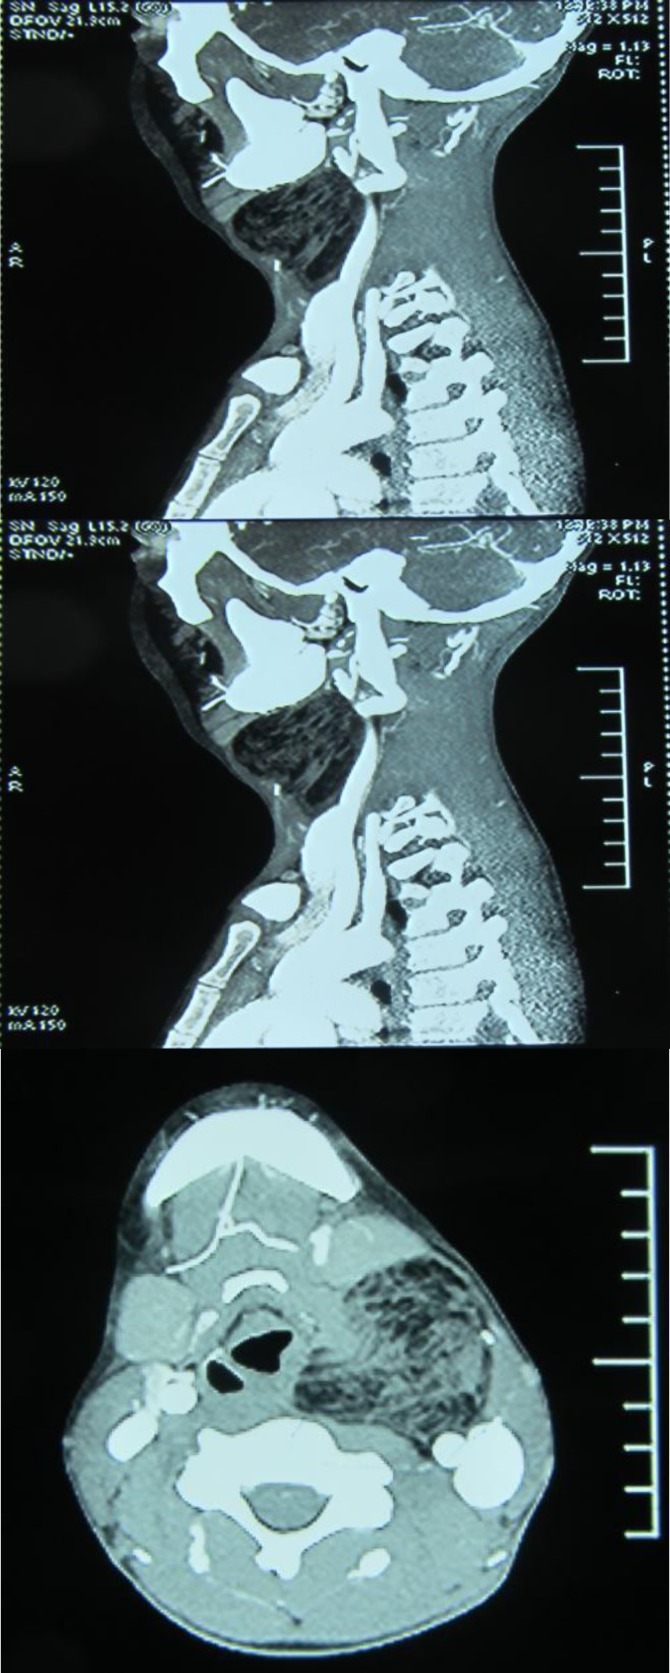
CT scan of neck with contrast (coronal, sagital, and axial view) revealed non-enhanced paraglottic space mass lesion
